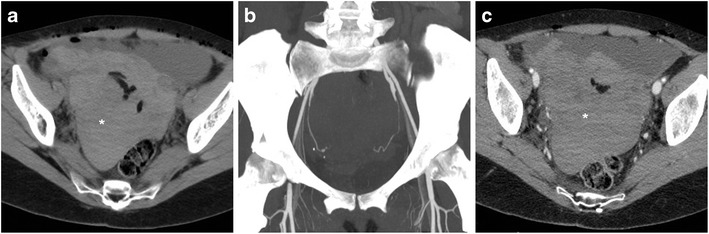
Large pelvic haematoma developing within 48 h after LH for uterine myoma, manifesting with hypotension and severe haemoglobin drop. Axial precontrast CT image (A) showed abundant, strongly hyperattenuating (60–70 HU) blood. CT angiography (maximum-intensity reformation B) and venous-phase CT acquisition (C) did not show active contrast medium extravasation suggesting active bleeding. Repeated laparoscopy was required for drainage of the peritoneal cavity and haemostasis
